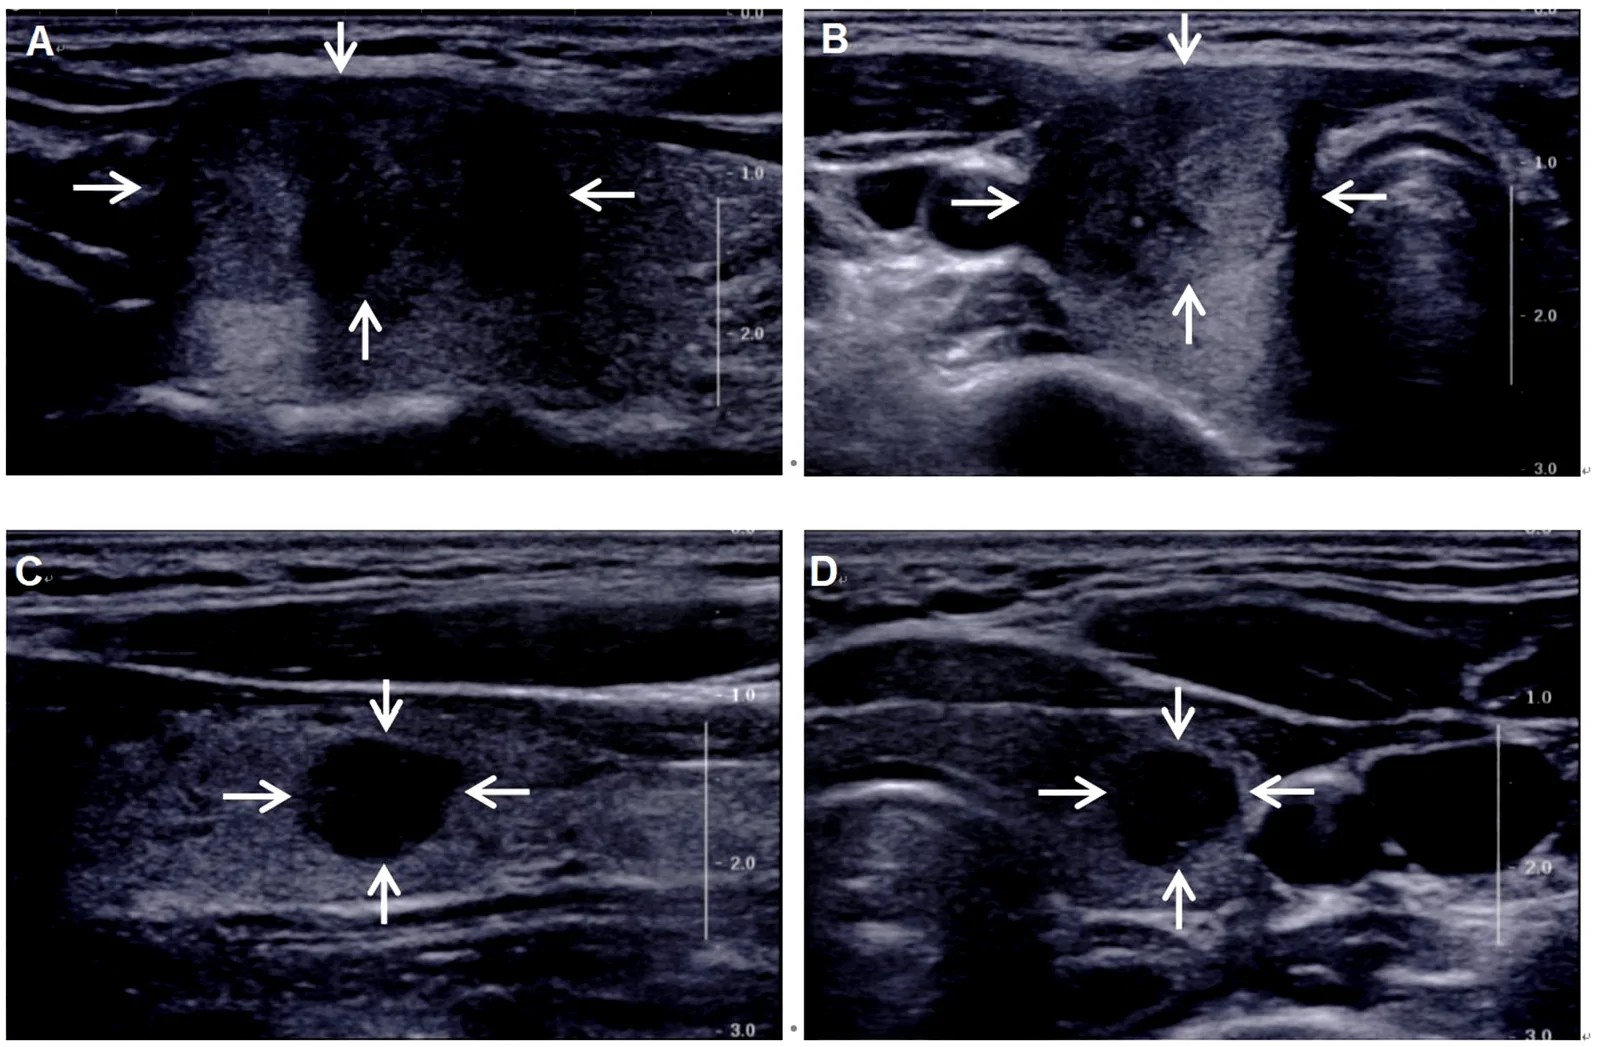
Ultrasound images comparing high versus low Ki-67 expression. (A, B): images from a patient with Ki-67 expression ≥ 5% (Case 1); (C, D): images from a patient with Ki-67 expression < 5% (Case 2). The lesion is indicated by white arrows.
